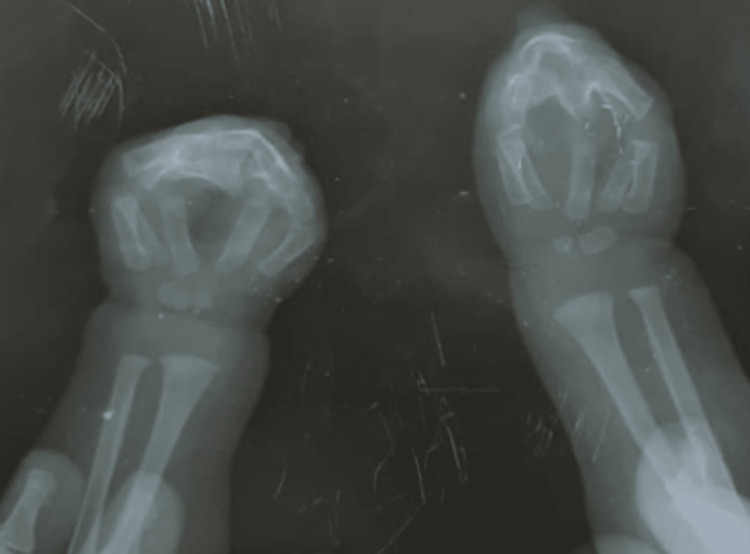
Hand-wrist radiograph showing soft tissue syndactyly and synostosis of the phalanges
